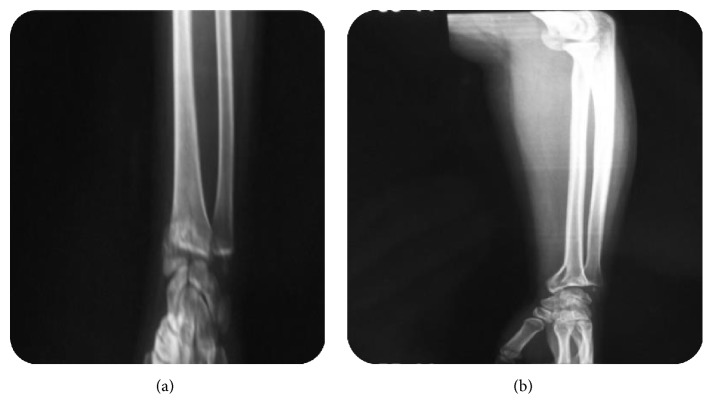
(a) Anteroposterior and (b) lateral radiographs showing comminuted articular fracture of the distal radius with an anterior displacement.
